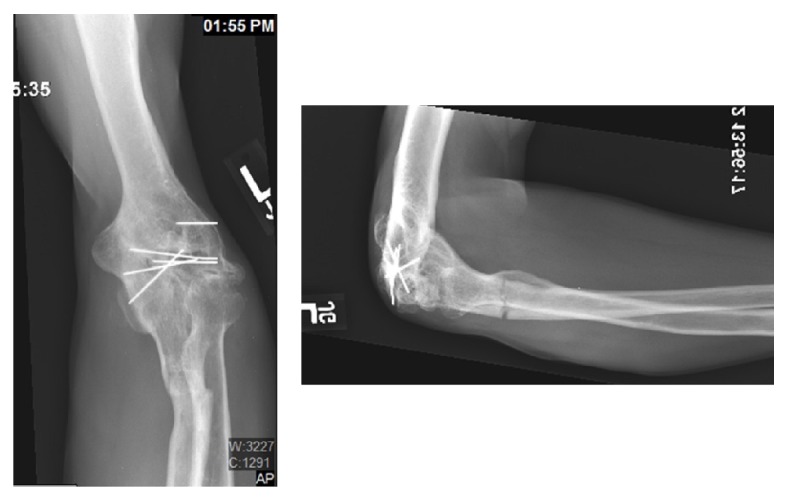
Plain radiographs of the elbow at the time of presentation revealed an elbow arthrodesis at 90 degrees with retained hardware and a minimally displaced transverse proximal ulnar shaft fracture.
